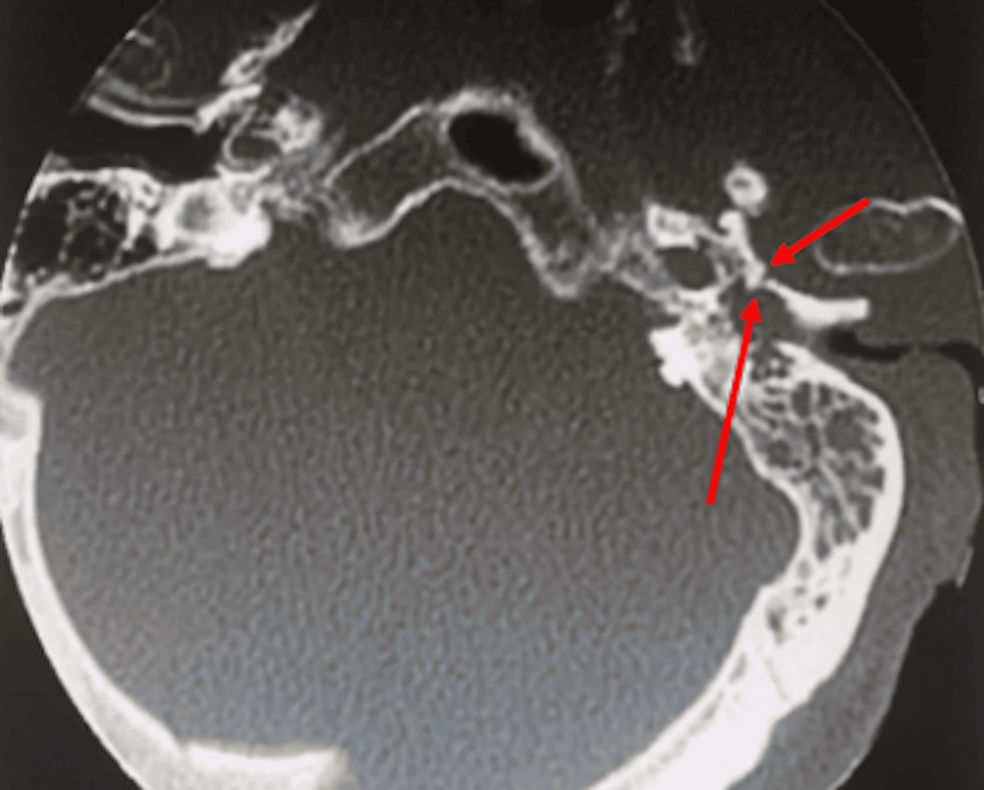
Cranial computed tomography Red arrows showing bone invasion of the mastoid cells.
